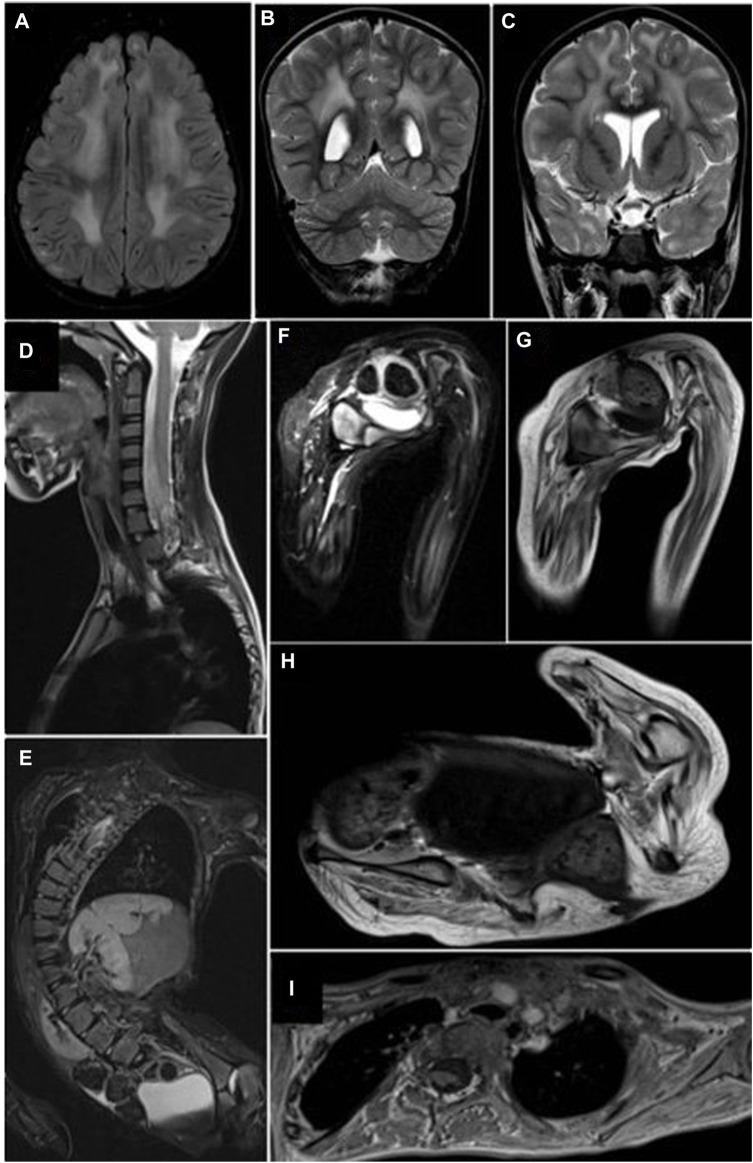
Cerebral and musculoskeletal system MRI. (A–C) Periventricular and subcortical white matter hyperintensities with a nonspecific demyelination pattern. FLAIR (A) and coronal T2 (B, C) sequences. (D, E) T2FS sequences. Thoracolumbar scoliosis secondary to muscular atrophy, without fusion defects or fragmentation of the vertebral bodies or compromise of the spinal canal. (F, G) Pelvis short tau inversion recovery (STIR) and T1 sequences. Diffuse and generalized muscular atrophy with Goutallier grade 3 fatty replacement without muscle edema. (H,I) T1 sequences of pelvic and shoulder girdle. Generalized decrease in muscle volume with Goutallier grade 3 fatty infiltration.
